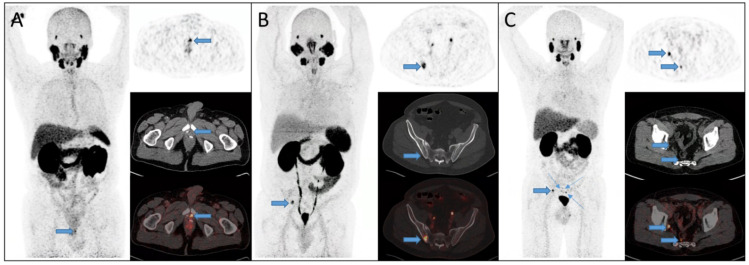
Patients with detected relapse on ^68^Ga-PSMA PET/CT. (A) A 75 year-old man with biochemical recurrence (PSA = 0.5 ng/mL) seven years after radical prostatectomy. Local relapse was visualized on ^68^Ga-PSMA PET/CT just anterior to the surgical clips (arrows). Subsequently, radiation therapy was performed on the relapse, and now four years later the patient has unmeasurably low PSA. (B) A 67 year-old male with biochemical recurrence (PSA = 5 ng/mL) after salvage radiation therapy. A bone metastasis in the right iliac bone was found (arrows). Subsequently the patient underwent stereotactic radiation therapy of the metastasis with currently two years free of progression. (C) A 59 year-old man with biochemical recurrence (PSA = 1.6 ng/mL) three years after radical prostatectomy. ^68^Ga-PSMA PET/CT showed small pelvic and mesorectal lymph node metastases (arrows).
